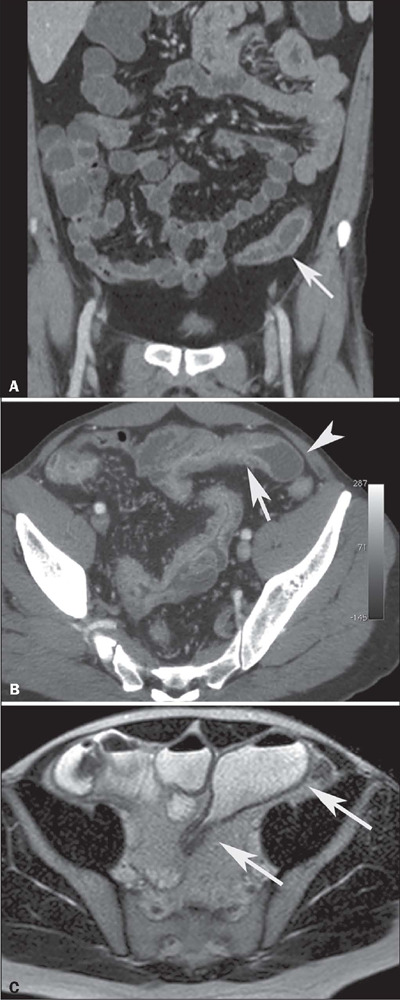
A: Coronal contrast-enhanced CT scan showing stenosis with mild mural thickening
